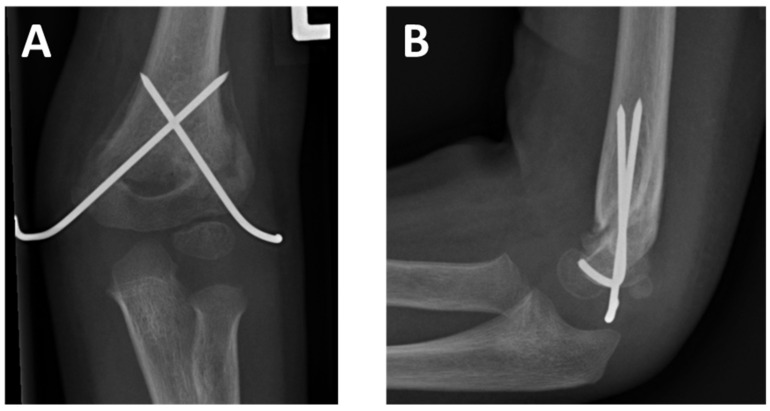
Radiograph of a supracondylar humeral fracture treated by percutaneous crossed pinning in anterior-posterior (A) and lateral view (B).
